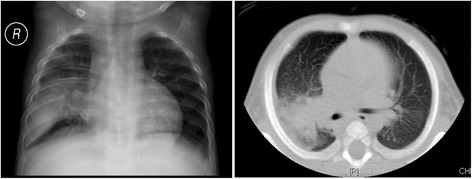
Chest radiograph and computed tomography scan of the patient at the age of 3 months, which revealed pneumonic infiltration and consolidation of the middle and lower lobes of the right lung
